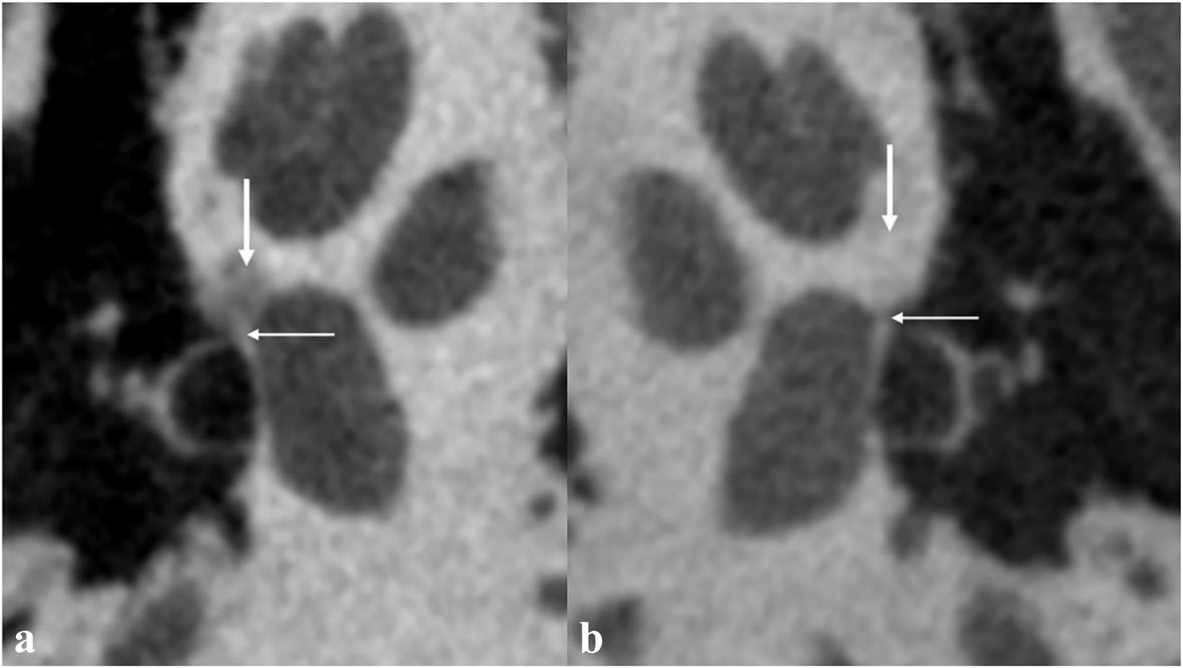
Typical U-HRCT imaging findings of fenestral otosclerosis in the otospongiotic phase. a Decreased bone density in the right fissula ante fenestram (thick arrow) and thickening of the right annular ligament (thin arrow). b Uniform bone density in the left fissula ante fenestram (thick arrow), and the left annular ligament is clearly shown with linear soft tissue density (thin arrow)
